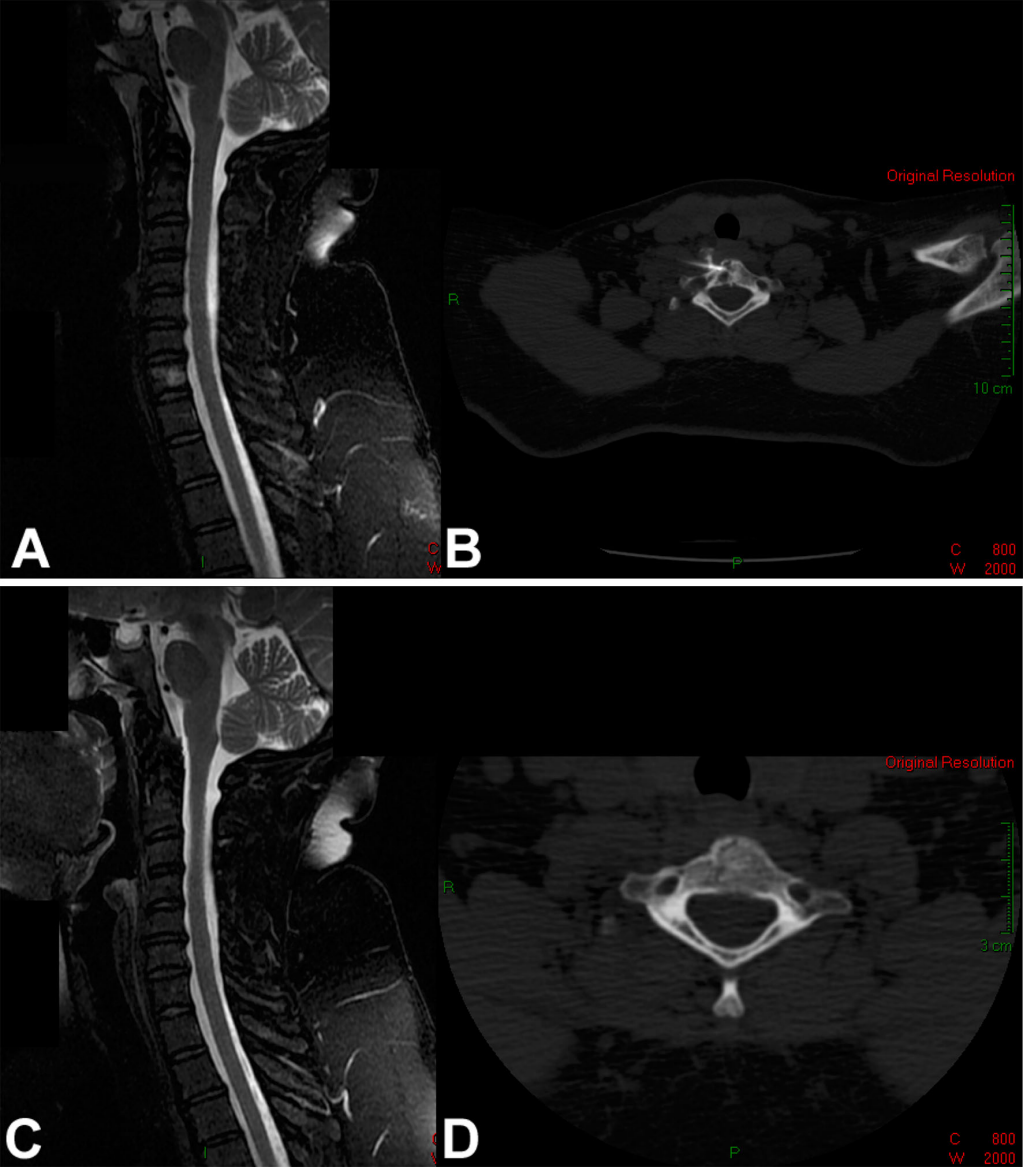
a Sagittal T2-weighted MRI with fat suppression of the cervical spine of a 43-year-old woman with a painful osteolytic lesion of the C7 vertebral body. b CT-guided frozen section biopsy showed eosinophilic granuloma; intralesional methylprednisolone injection was performed. c Sagittal T2-weighted MRI with fat suppression. d Axial CT scan show complete reconstitution of the lesion 4 years after diagnosis and treatment
